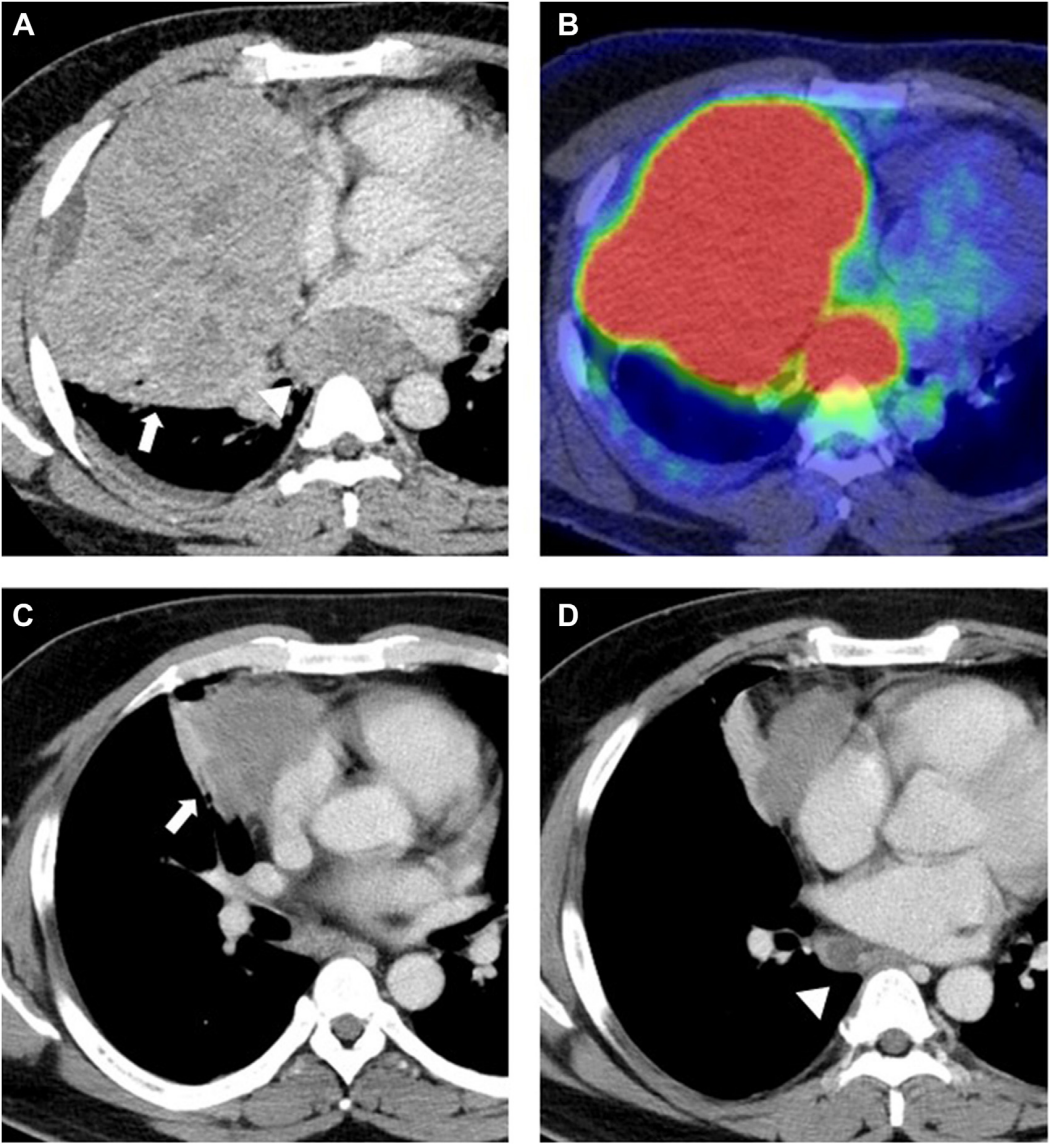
Preoperative radiographic findings. (A) Contrast-enhanced computed tomography (CT) revealing a mediastinal tumor protruding into the right thoracic cavity (arrow) and enlarged subcarinal lymph nodes (arrowhead). (B) Positron emission tomography/CT revealing a high fluorodeoxyglucose uptake (28.7 and 22.4 in the main tumor and subcarinal lymph nodes, respectively). (C, D) CT revealing shrinking mediastinal tumor (arrow) and subcarinal lymph nodes (arrowhead) after chemotherapy.
